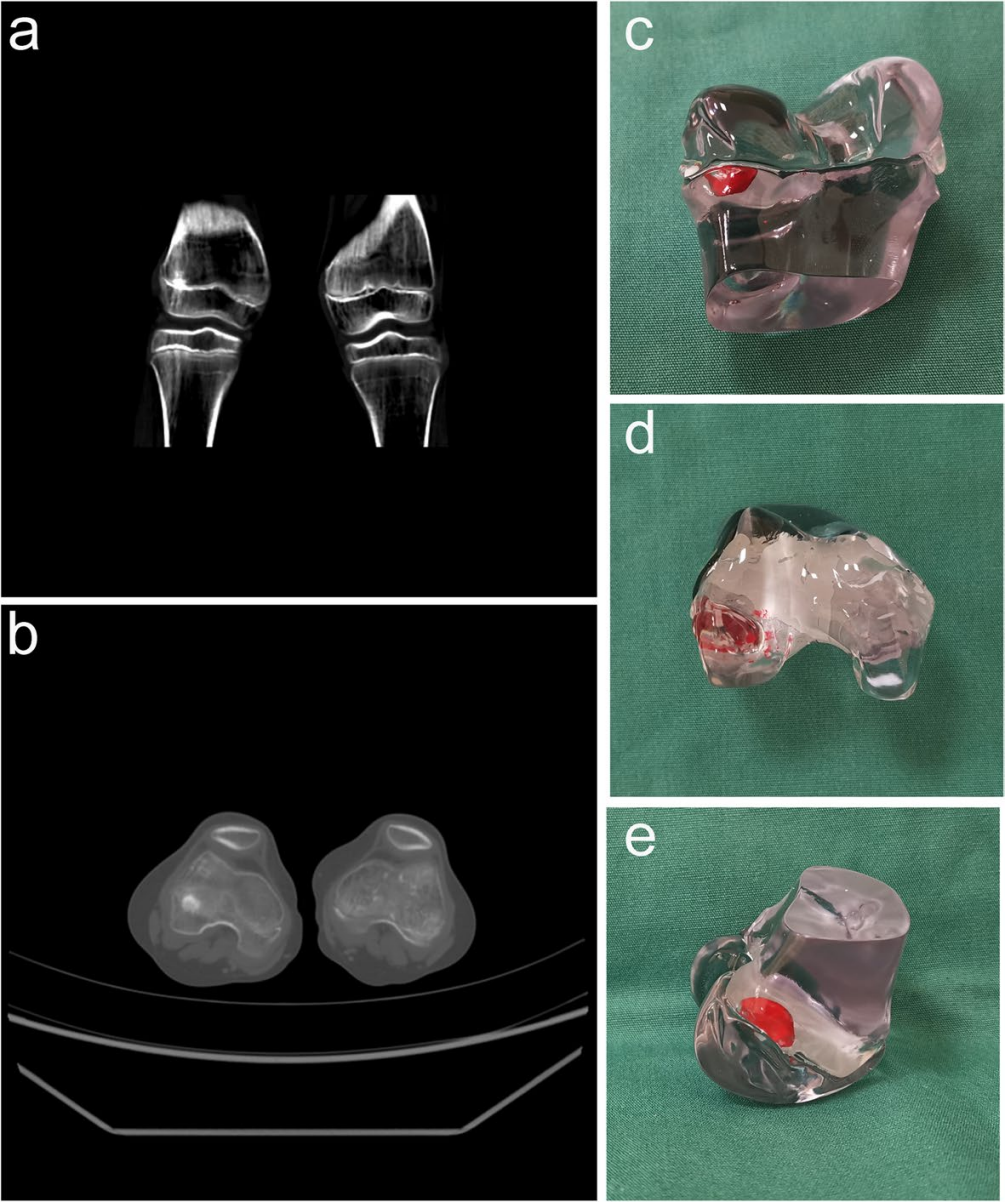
The visualization of the physeal bar in CT and 3D printing model. The coronary view (a) and horizontal view (b) in CT; The posterior-anterior view (c), up-down view (d), and lateral-medial view (e) of the model. The red part represents the physeal bar
